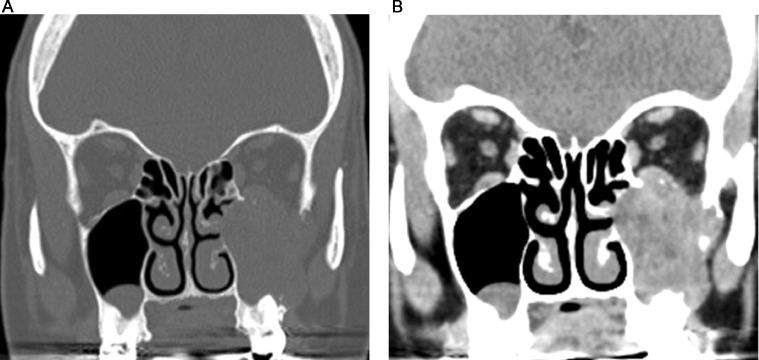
A. Coronal CT bone window: Non-calcified left maxillary sinus mass, with bony erosion along the left orbital floor and lateral wall of the left maxillary sinus, B. Coronal CT soft tissue window: Left maxillary sinus mass, with intra-orbital extension superiorly and buccal space laterally.
